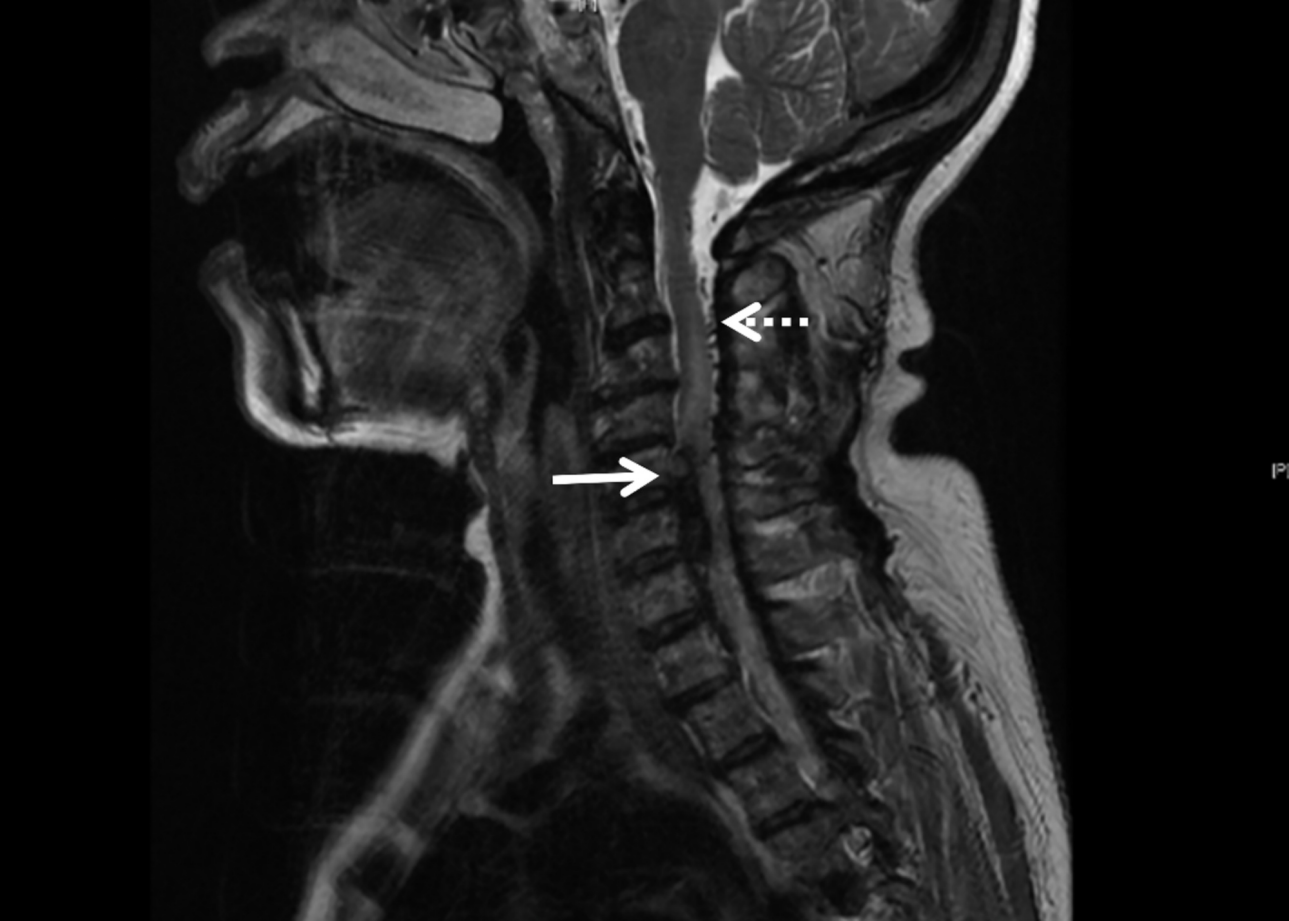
Sagittal magnetic resonance imaging (MRI) shows a C5-C6 spinal hematoma (solid arrow), and abnormal vessels adjacent to the dorsal aspect of the cervical canal (dotted arrow)
